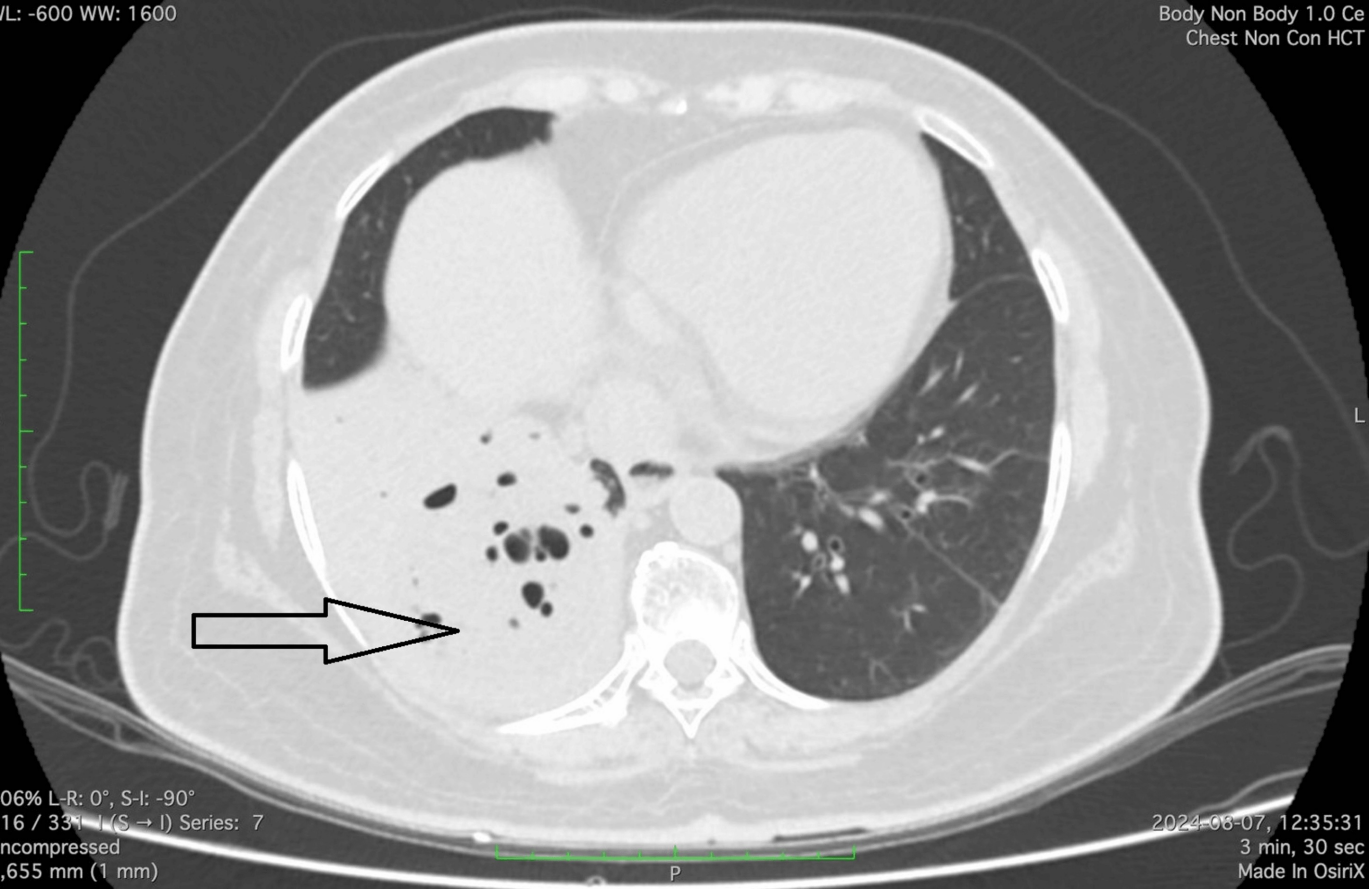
High-resolution CT of the chest revealing right lower lobe consolidation with central necrosis (black arrow), consistent with necrotizing pneumonia.
